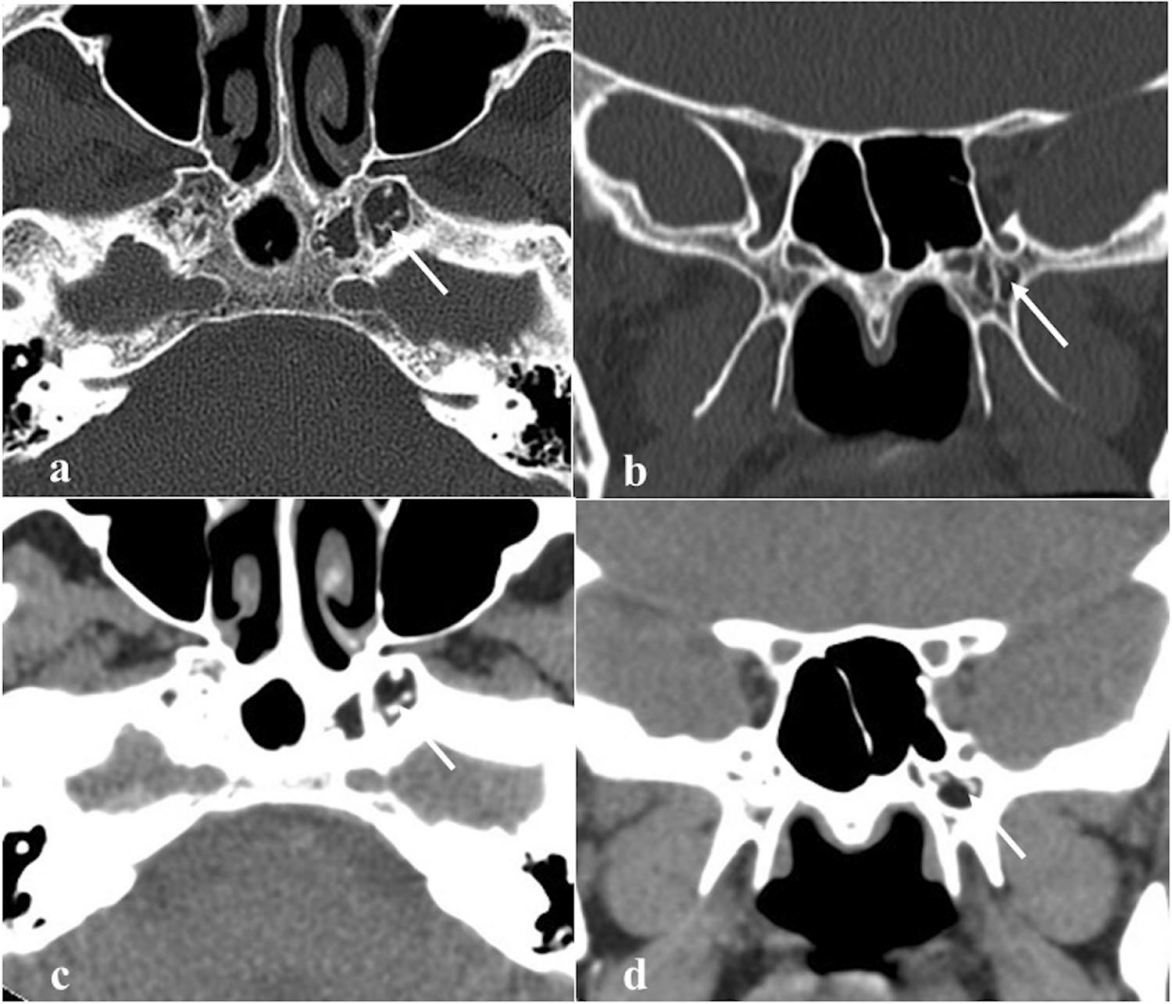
Sphenoid haemangioma as an incidental finding in a 70-year-old female patient with sarcoidosis and chronic rhinosinusitis. CT shows a small osteolytic lesion (white arrows) in the left greater sphenoid wing characterised by well-defined sclerotic margins and a “sunburst appearance” on axial (a) and coronal (b) images. The fatty component is found on axial (c) and coronal (d) soft tissue reconstruction algorithm images
